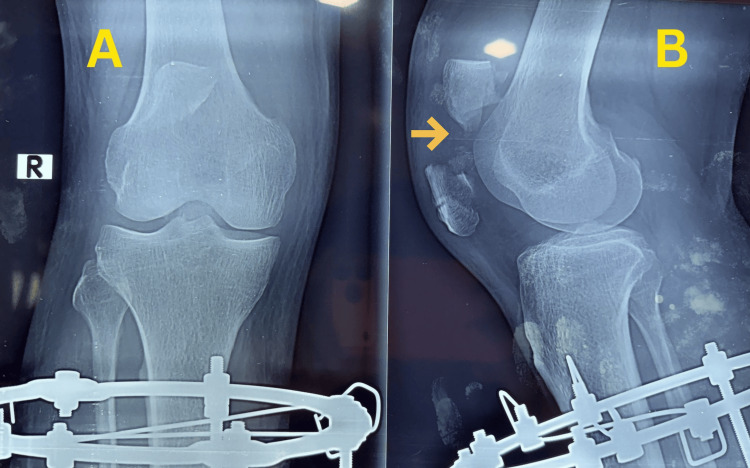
Radiograph showing delayed diagnosis of patellar fracture Knee radiographs obtained after surgery demonstrating the associated patellar fracture: (A) anteroposterior view and (B) lateral view. The arrow indicates the patellar fracture site.
